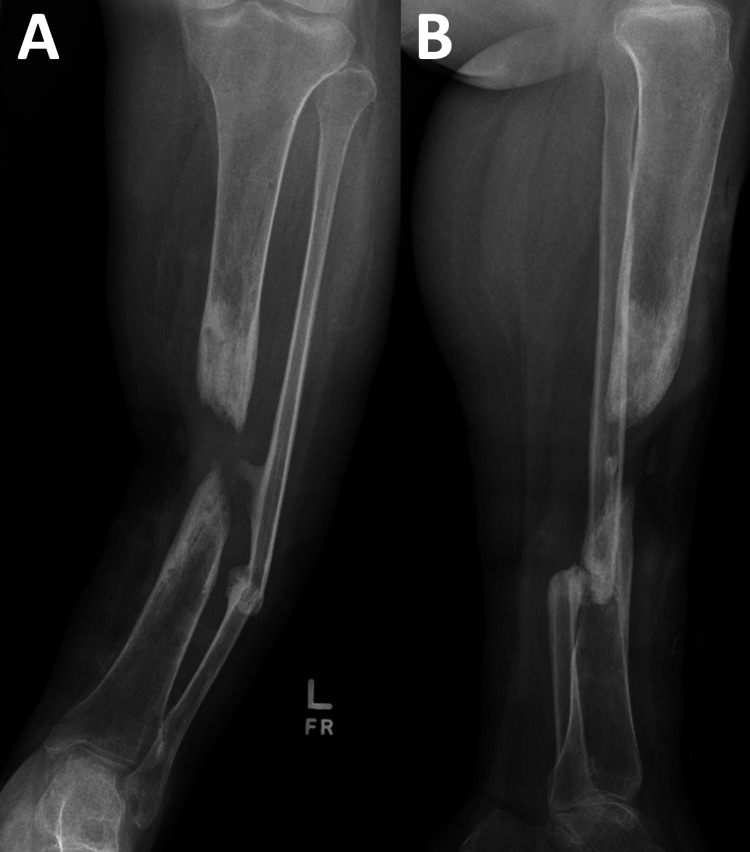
(A) Anteroposterior and (B) lateral radiographs of a left tibia non-union demonstrating a significant bony defect with varus malalignment and diffuse osteopenia.
